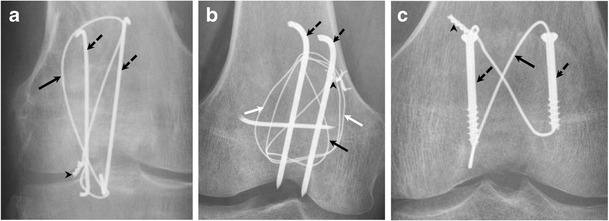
Frontal knee radiographs showing examples of common patellar fracture fixation constructs using tension band wiring. (a) Modified anterior tension band (MATB) technique (also called K-wire technique) with vertical figure eight loop configuration and wire twist. (b) MATB with vertical figure eight and wire twist in addition to cerclage. (c) Cannulated screws with horizontal figure eight loop
